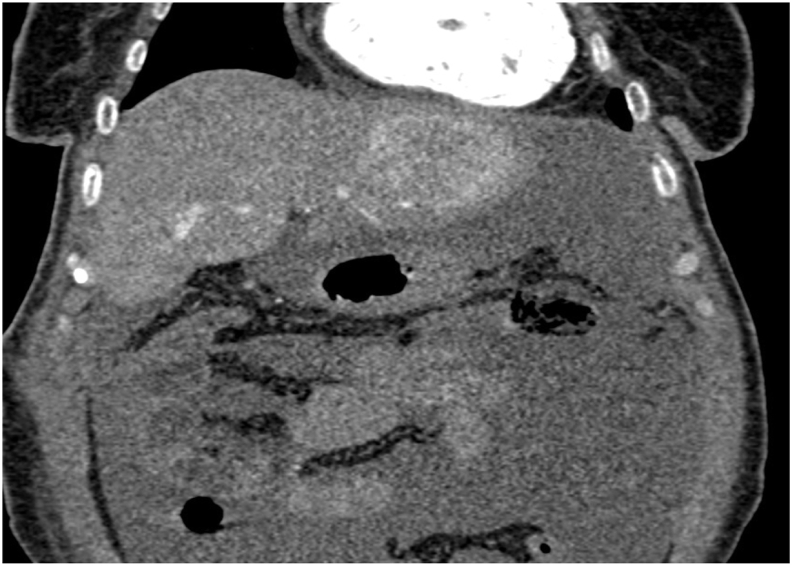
A CT scan revealed a voluminous soft mass of the left liver with exophytic development.
